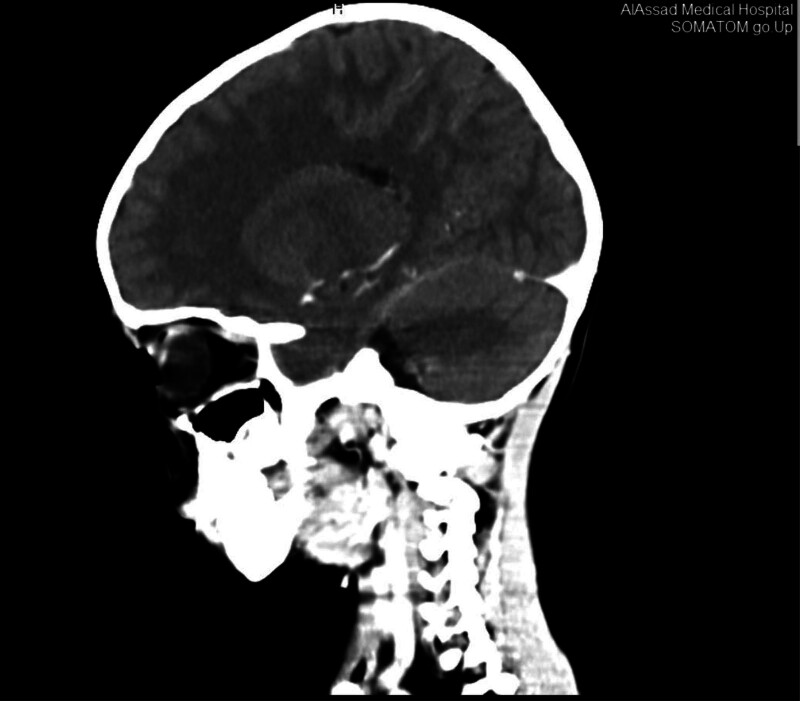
is a cranial CT that shows a lesion in the Sella turcica with extension into the optic chiasm and sphenoid bone. CT = computed tomography.
